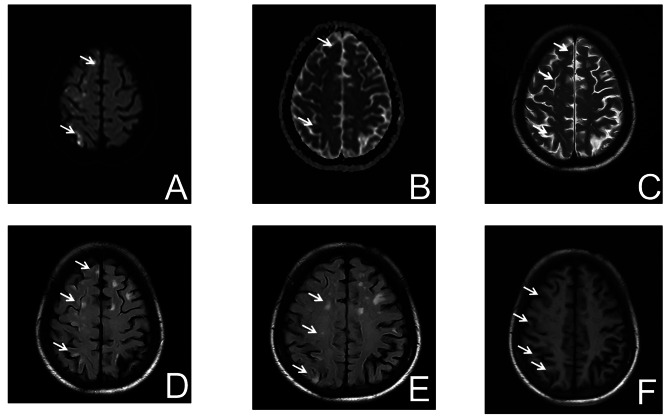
Brain MRI findings. A: DWI head MRI; B: ADC head MRI; C: T2-weighted head MRI; D: Flair head MRI; E ,F: T1-weighted head MRI
